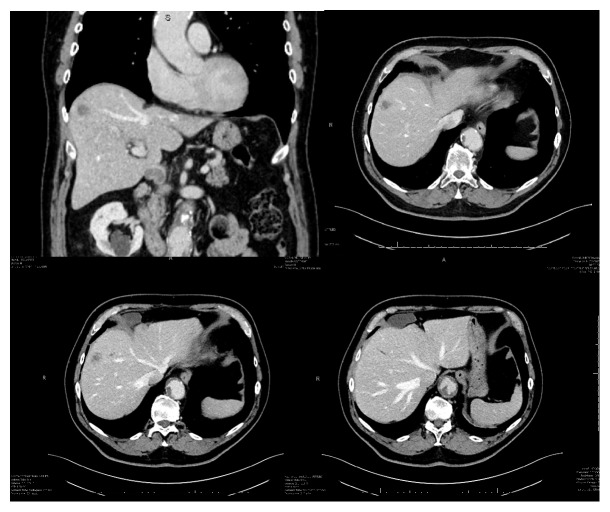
Preoperative CT imaging of hepatic lesion.
